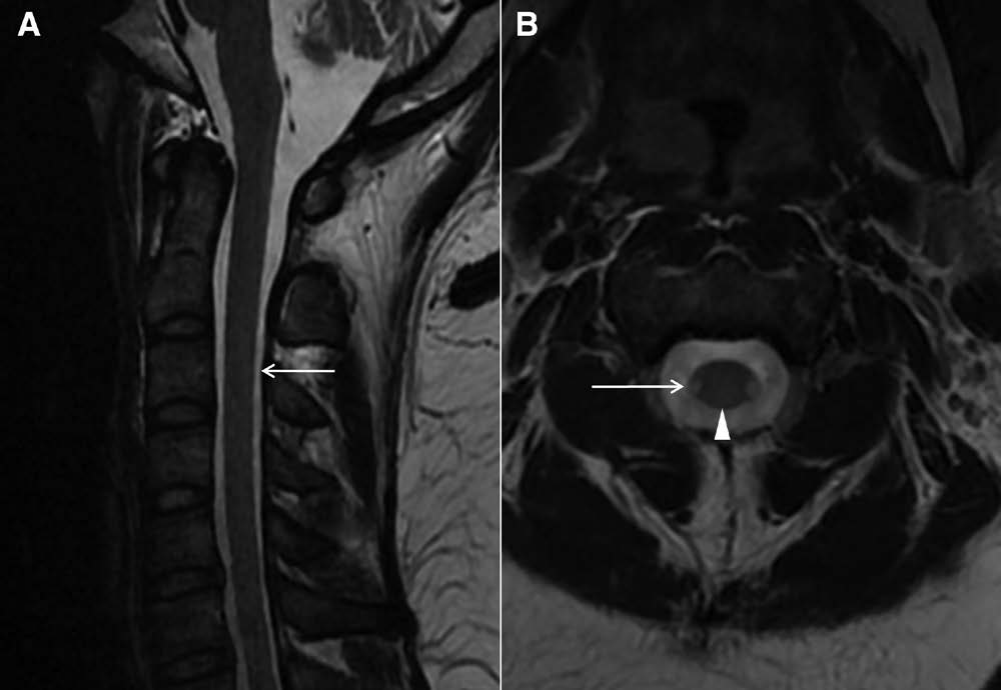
MRI 7 mo later. MRI of cervical cord shows disappeared hyperintense signal in posterior column (A, white arrow; B, triangular arrow). Axial T2 image indicates hyperintensity in the lateral column of the cervical cord (B, white arrow). MRI = magnetic resonance imaging.
